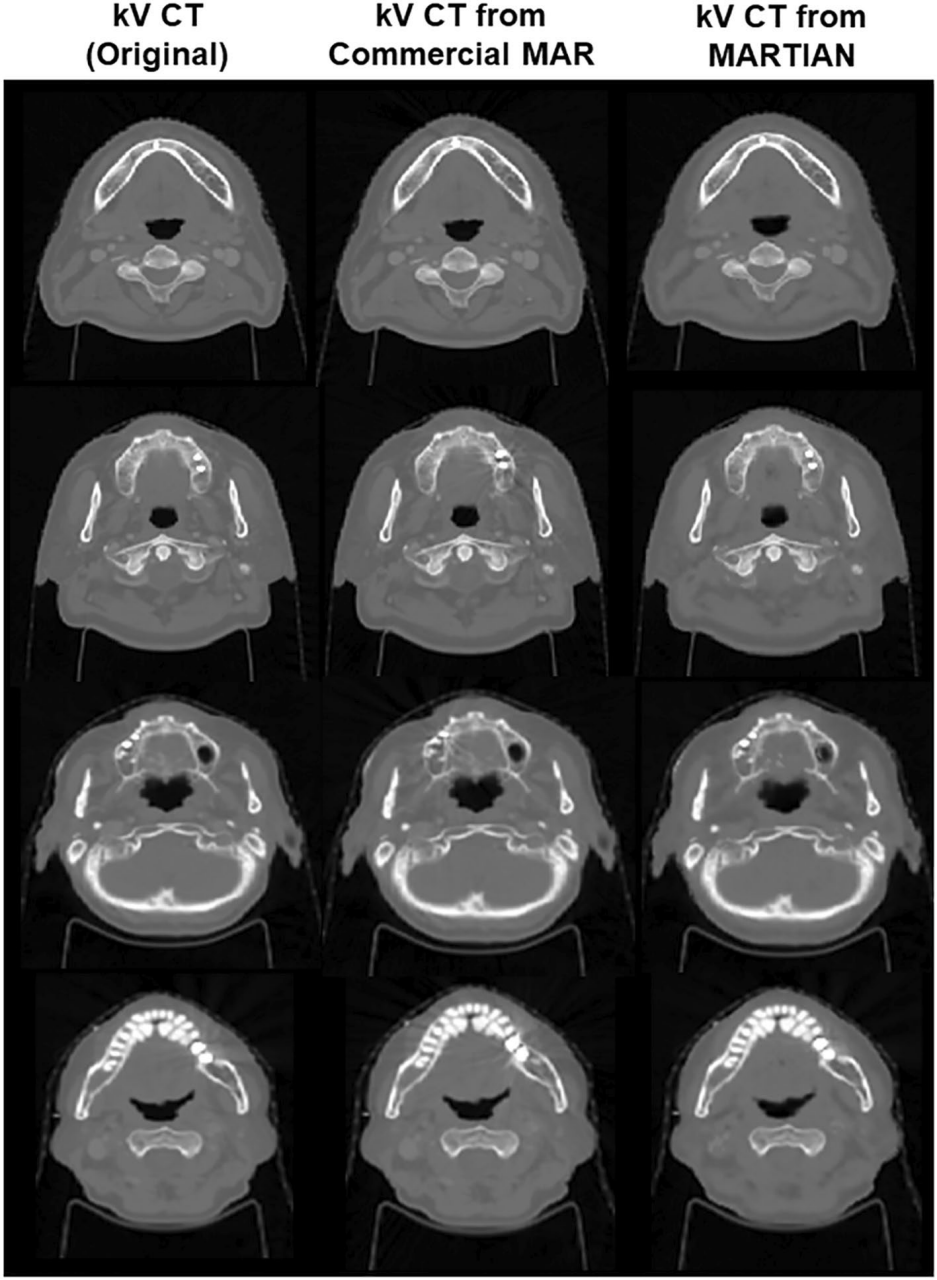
The predicted kV CT images from the proposed (third row) for 2D slices with high density (HU > 4000) and no containing metal-contaminated artifacts as seen in the true kV CT images (first row), and kV CT from the commercial MAR program (kV CT: [−1000 HU, 1500 HU]).
